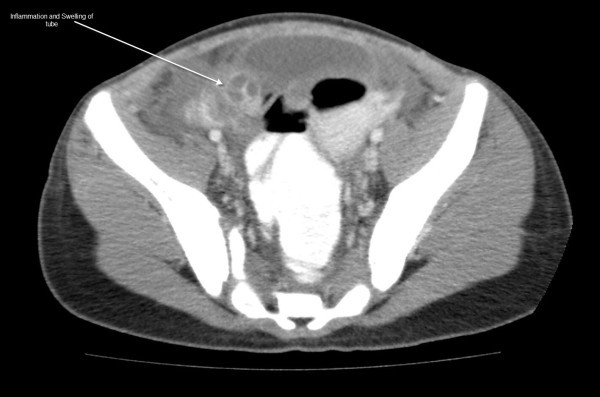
CT scan of the abdomen and pelvis with intravenous and oral contrast. A lower cut on the CT scan demonstrating extension of inflammation.
